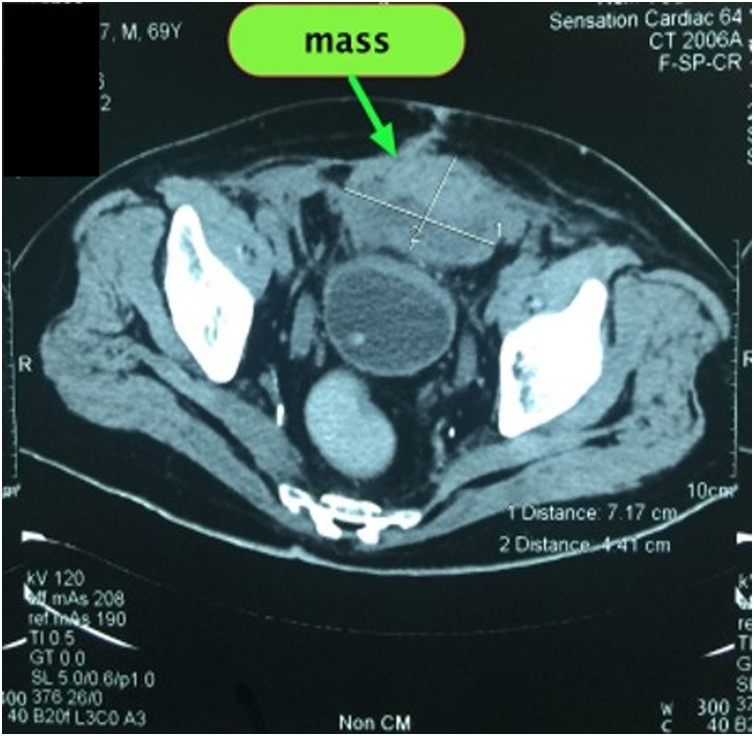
3-month follow-up CT Scan sagittal view showed mass in the abdomen.
